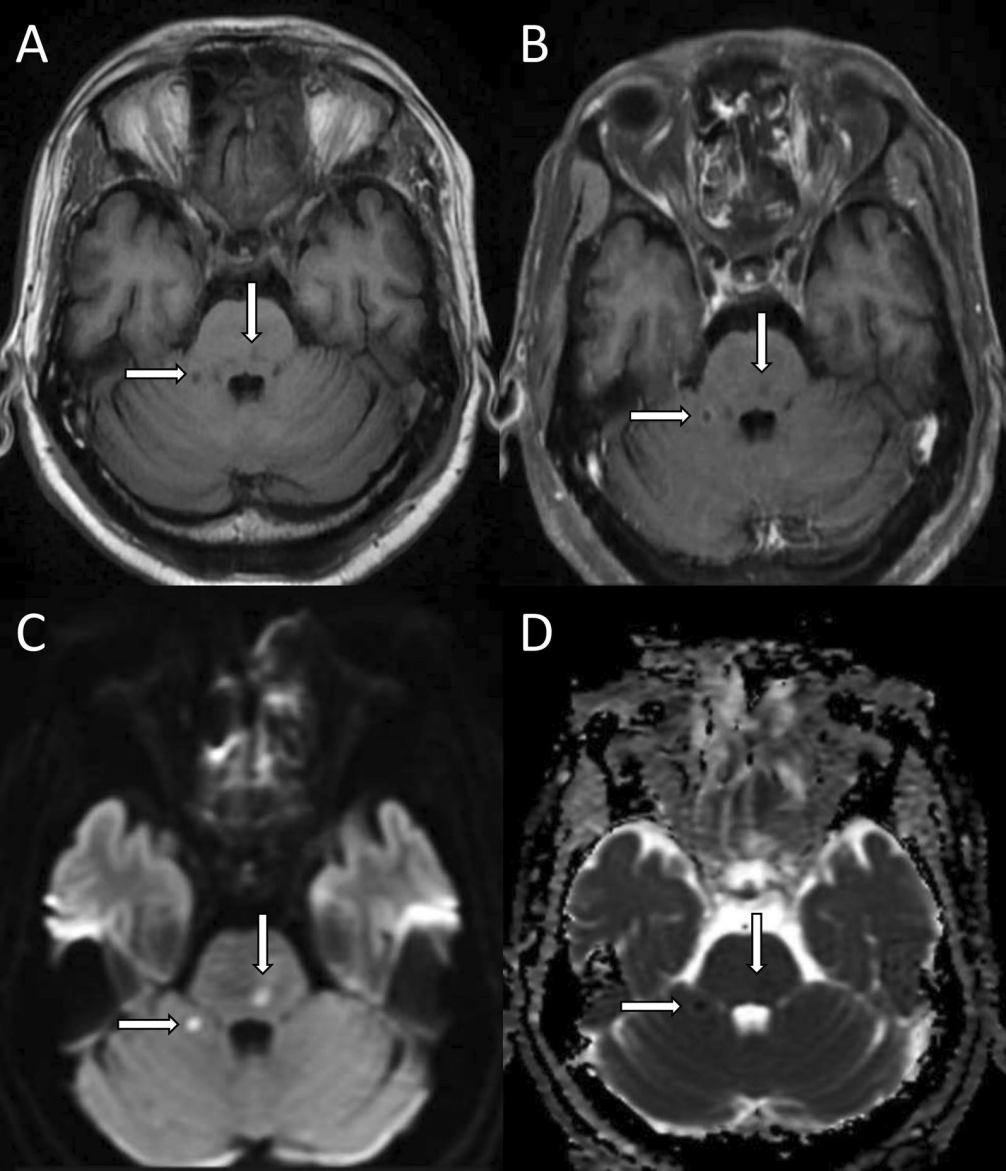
Septic emboli. Axial T1-weighted (A) and contrast-enhanced fat-saturated (B) images show lesions in the right pontocerebellar junction and pons with peripheral enhancement (white arrows). Diffusion-weighted and apparent diffusion coefficient (ADC) images (C, D) show foci of diffusion restriction (white arrows). In a known case of mucormycosis features indicate septic emboli
